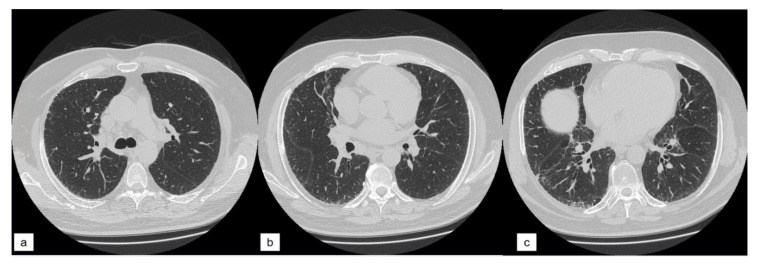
The three zones of high resolution computed tomography (HRCT) visual score. (a) “upper zone”, slice at the level of carina; (b) “middle zone”; (c) “lower zone” slice at the level of the inferior pulmonary vein.
